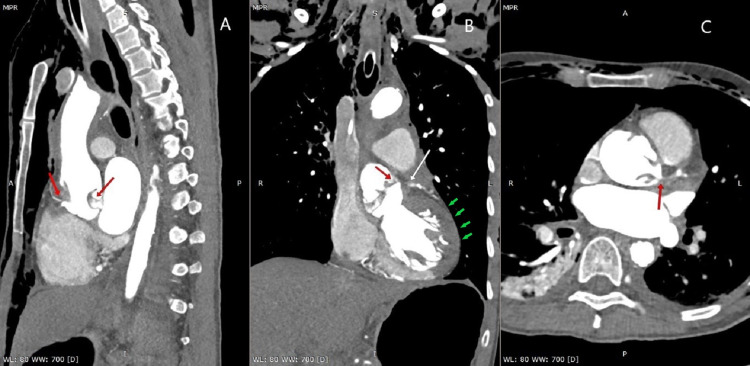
Contrast CT aortogram [A] sagittal view showing the dissection flap originating anteriorly near the right coronary artery ostium (red arrow) and extending posteriorly and attached near the LMCA ostium (red arrow), [B] coronal section showing the dissection flap (red arrow) causing complete occlusion of the LMCA (white arrow) with nonenhancement of the myocardium (green arrows), [C] cross-sectional view showing the dissection flap obstructing the LMCA (red arrow) and faint filling of the branches of the LMCA. LMCA- left main coronary artery, CT- Computerized tomography
